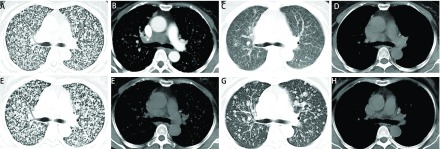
患者治疗过程中胸部影像学的变化情况。A、B：2018年3月28日胸部CT示双肺弥漫结节，纵隔及右肺门淋巴结增多、增大；C、D：2018年5月14日经吉非替尼治疗1个月后复查胸部CT示与2018年3月28日胸部CT比较，双肺结节减少、缩小；E、F：2018年10月1日再次复查胸部CT示与2018年5月14日胸部CT检查比较，双肺结节明显增多；G、H：2018年11月30日复查胸部CT示与2018年10月1日胸部CT检查相比，双肺结节减少。 Changes of chest imaging in the patient during treatment. A, B: Chest CT shows (March 28, 2018) diffuse nodules in both lungs, mediastinal and right hilar lymph nodes increased in number and size; C, D: After Gefitinib treatment for 1 month reexamination of chest CT (May 14, 2018) shows decreased lung nodules zoom out comparing with chest CT on March 28, 2018; E, F: Reexamination of chest CT (October 1, 2018) shows bilateral lung nodules were obvious increased in number comparing with chest CT on May 14, 2018; G, H: Chest CT (November 30, 2018) shows bilateral pulmonary nodules decreased comparing with chest CT on October 1, 2018. CT: computed tomography.
